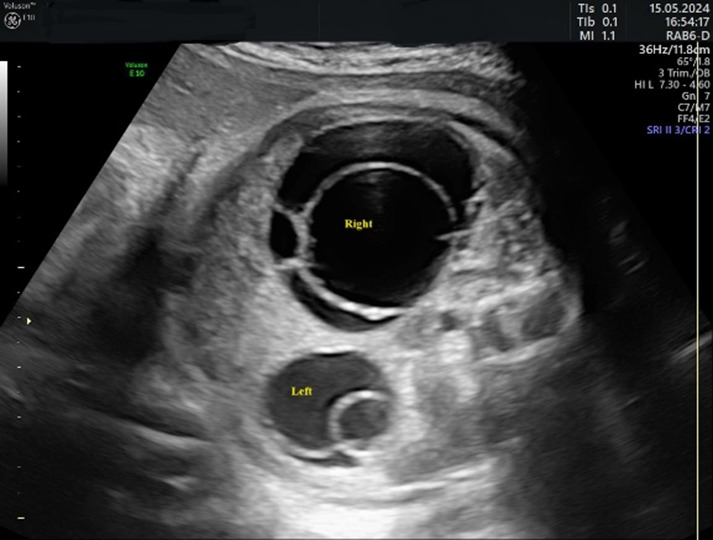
Ultrasound axial view. Simple ovarian cysts in the 33rd week of pregnancy. Smaller cysts (arrows) are daughter cysts that are present on both sides.
